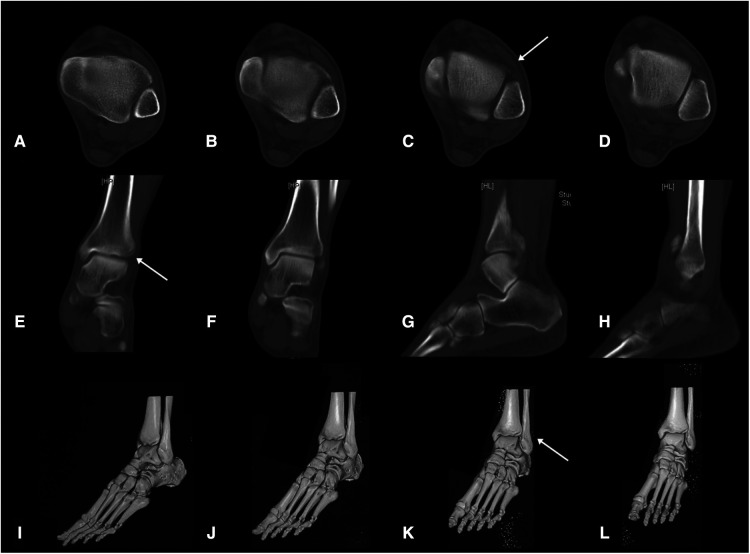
The initial transverse view (A–D), coronal view (E,F), and sagittal view (G,H) CT images of the ankle, and 3D reconstruction image of the foot and ankle (I–L).
